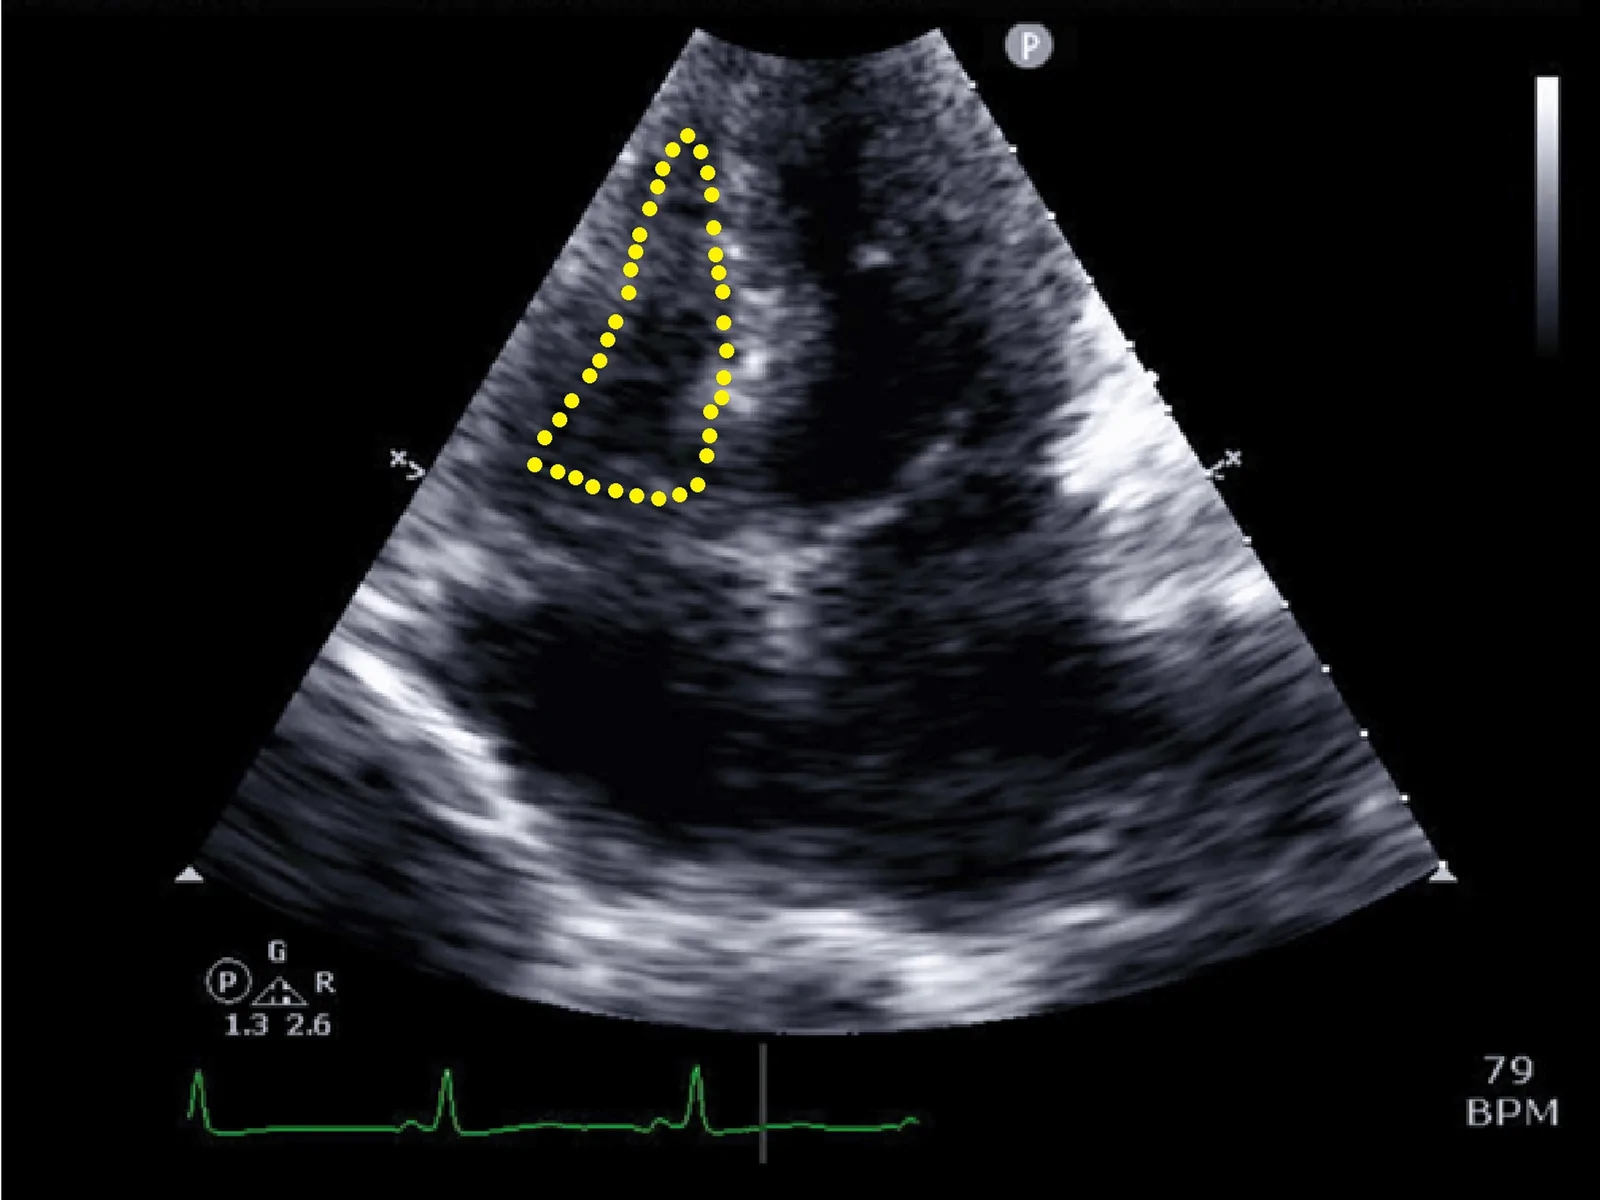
Two-dimensional transthoracic echocardiography Apical four-chamber view showing air bubbles as numerous hyperechoic foci within the right ventricle cavity (roughly outlined by a yellow dotted line), as opposed to the anechoic nature of the other heart chambers.
